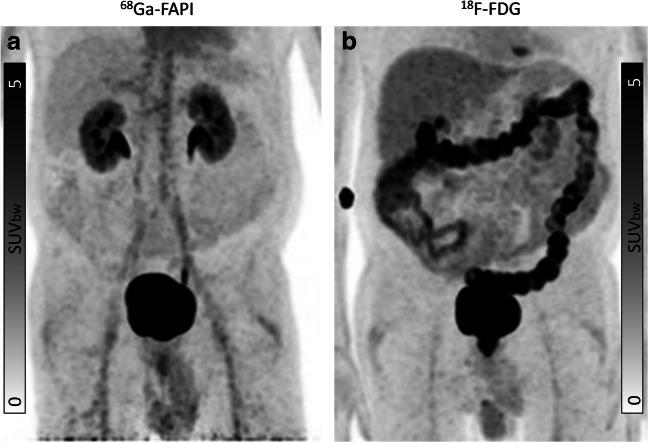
Intra-individual comparison of ^18^F-FDG and ^68^Ga-FAPI in a patient with oral squamous cell carcinoma presenting with inflammation in the colon as incidental finding in the ^18^F-FDG PET/CT (b), while no acute inflammatory process is associated with a ^68^Ga-FAPI-positive PET signal (a). The direct quantitative comparison presents a high SUVmax of 8.11 in the ^18^F-FDG PET/CT, while ^68^Ga-FAPI PET/CT demonstrates low tracer accumulation (SUVmax: 0.38)
